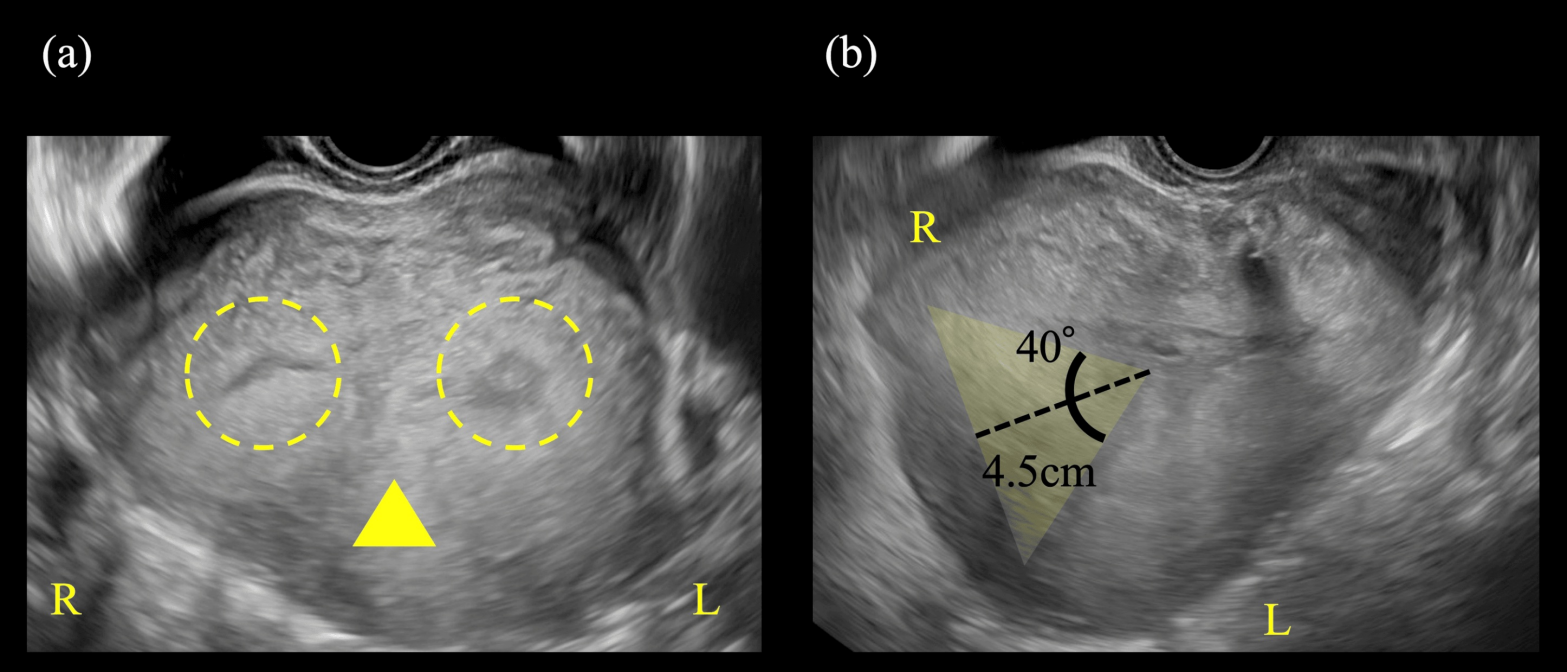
Predischarge transvaginal ultrasonography image of the uterus (a) Predischarge ultrasonography image of the uterus shows a septum (arrowhead) dividing it into two cavities (dotted circle). (b) The uterine fundus has a planar shape. The septum measured 4 cm in length with a septal angle of 40°, and no serosal indentation was observed
